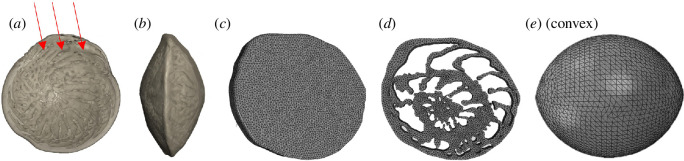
Image of the examined species *A. lobifera* rendered from a CT scan in spiral (*a*) and side (*b*) views, and models used for FEA representing the external (*c*) and internal (*d*) structures, and overall convex shape (*e*). Arrows represent force direction applied on the models for FEA.
